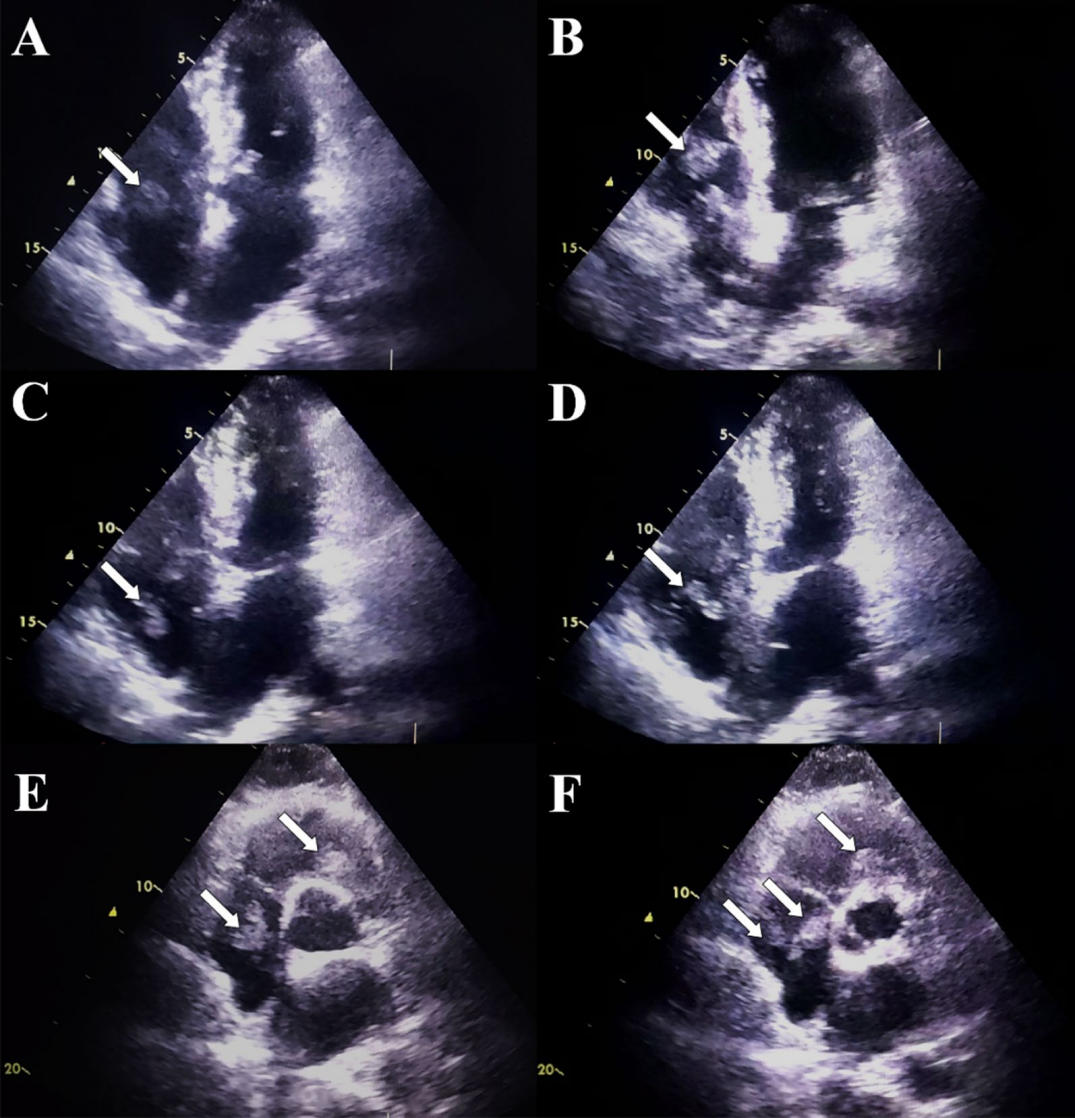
Transthoracic echocardiography of the patient in the apical four-chamber view (A, B, C and D) and the parasternal short axis view (E and F) in diastole (A, B and E) and systole (C, D and F) showing the presence of several centimetric emboli floating in the right atrium and ventricle (white arrows)
